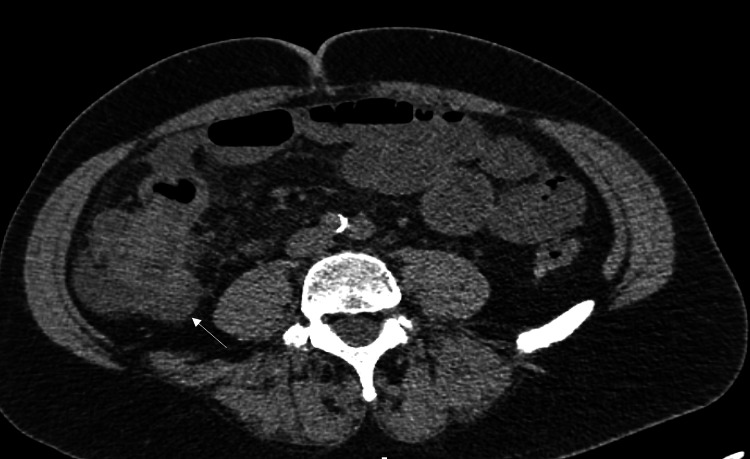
CT abdomen, axial cut, cecal mass (arrow)
